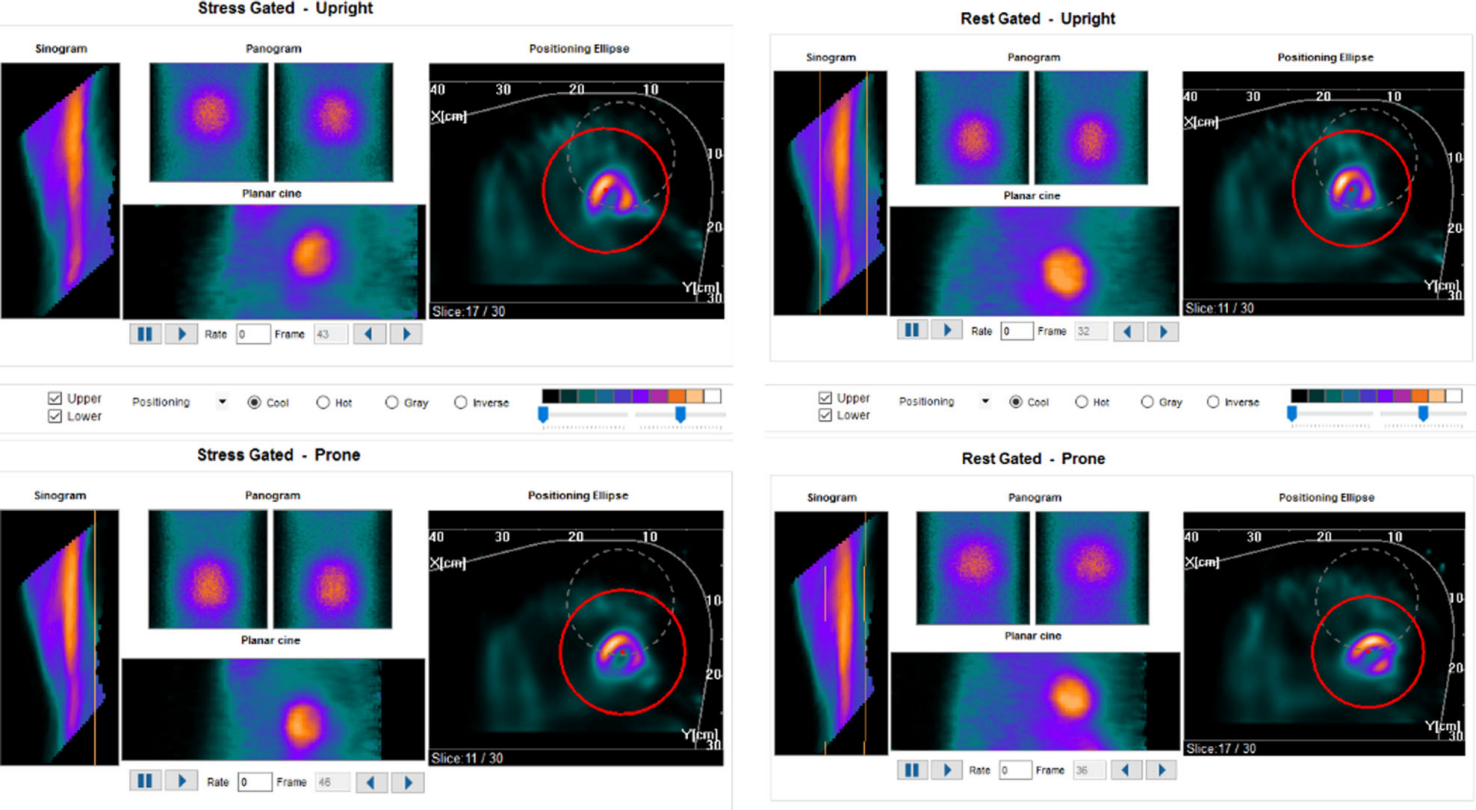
Data quality control report from the D-SPECT with the upright position at the top row and the prone position at the bottom row, stress and rest. The sonogram and panogram shows no movement during the scans. In the image named positioning ellipse the distance between the detector arm and the left ventricle is presented and the left ventricle has unchanged relation to the detector arm at both stress and rest
